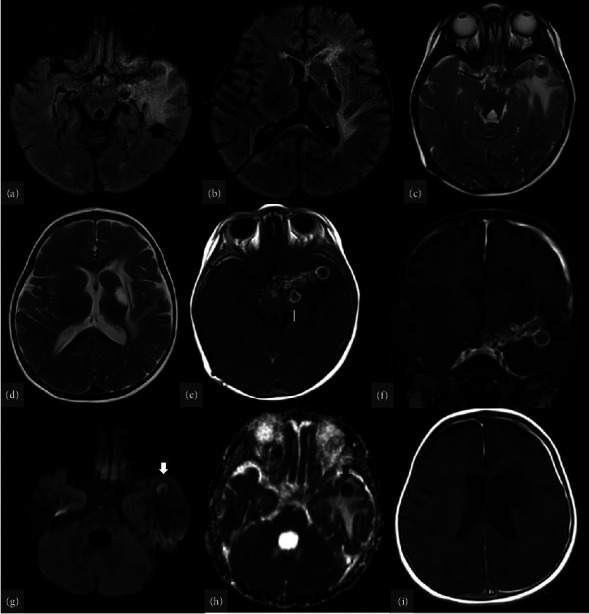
Follow-up MRI with various sequences. T2W/FLAIR sequences (a–d) reveal vasogenic edema in the left frontotemporal lobes, causing ventricular compression. Some round lesions are also visible, which appear hyperintense on T1W and isointense to the cortex on T2W. Following contrast administration (e, f) leptomeningeal enhancement and rim-enhancing nodules appear in the prepontine cistern and perisylvian fissure. Central diffusion restriction is seen on DWI/ADC (g, h), along with thickened pachymeningeal enhancement in the left frontoparietal region (i).
